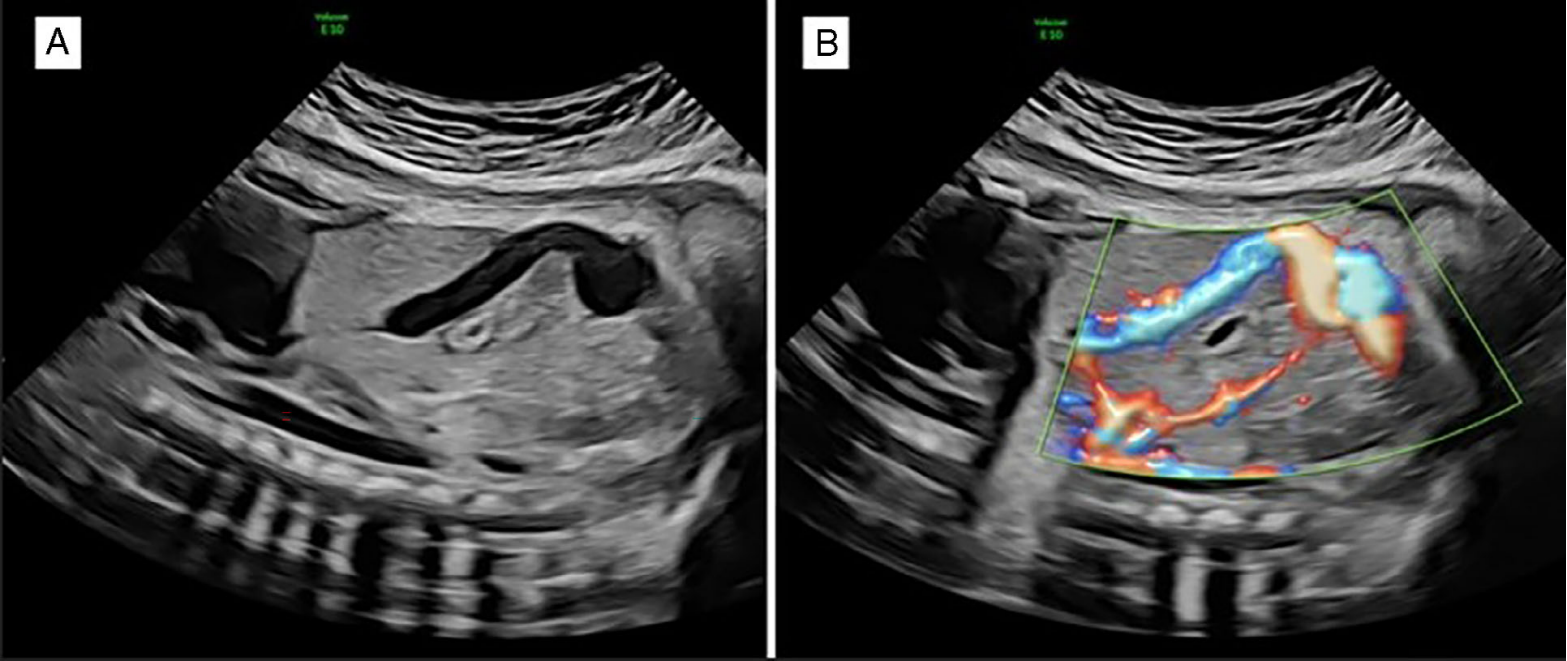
Fetal intra‐abdominal umbilical vein varix on 2D ultrasound (A) greyscale (B) with power doppler.
